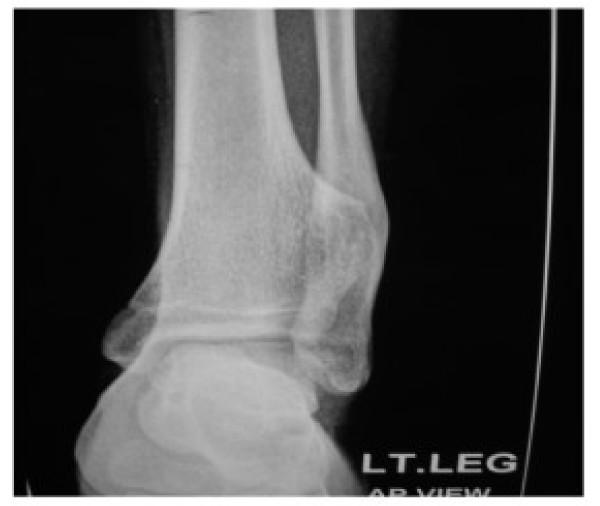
AP radiograph of ankle of a patient showing well defined exostosis arising from interosseous border of tibia pushing fibula.
